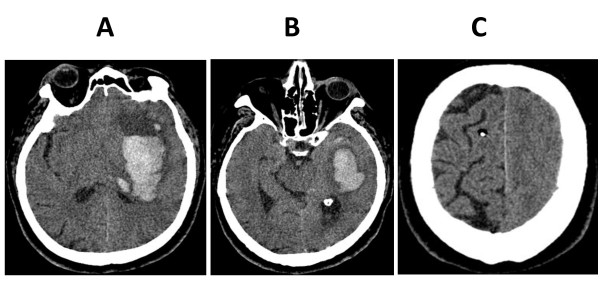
CT scan images after clinical signs of brain herniation developed. (A) Basal ganglia hemorrhage with perifocal edema. (B) Left-sided transtentorial herniation of the parahippocampal gyrus. (C) Edema of the left hemisphere with normally appearing right hemisphere.
